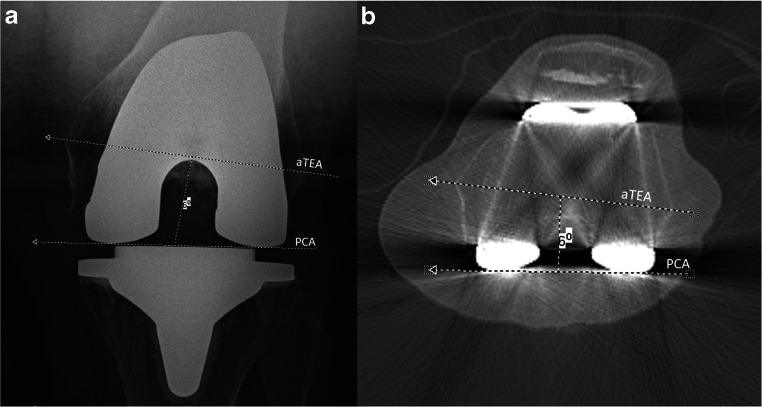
The anatomical posterior condylar angle formed by the anatomical transepicondylar axis (aTEA) and the posterior condylar axis (PCA) on Kanekasu view radiograph (a) and 2D axial computer tomography (b) of a 59-year-old woman after total knee arthroplasty
